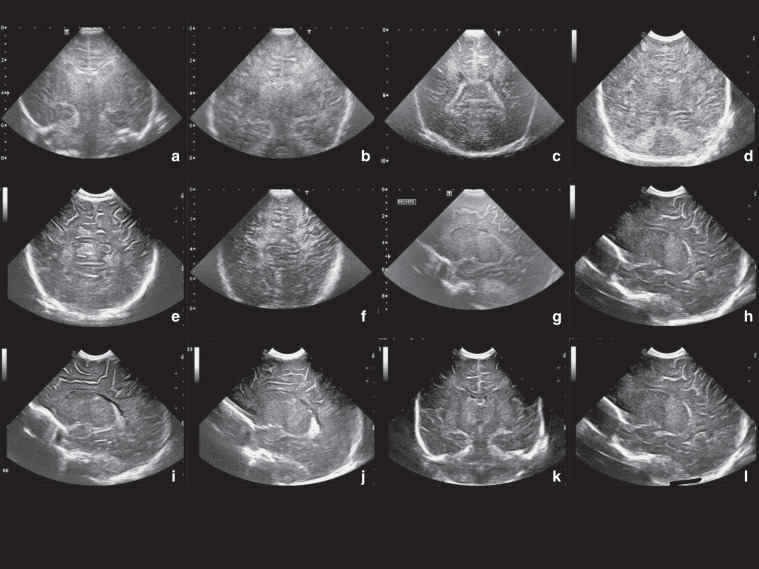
Cerebral ultrasound, outcome and HIE: example images. a Moderate cerebral oedema (1 point), b severe cerebral oedema (2 points), c moderate periventricular white matter (1 point), d severe periventricular white matter (2 points), e moderate subcortical white matter (1 point), f severe subcortical white matter (2 points), g moderate thalamus (1 point), h severe thalamus (2 points), i moderate putamen (1 point), j severe putamen (2 points), k “four-column sign” which means that both left and right thalamus and putamen are visible at the coronal view as four columns (1 point), l visibility of the PLIC (1 point). For the scoring sheet and definitions, see Table 1.
